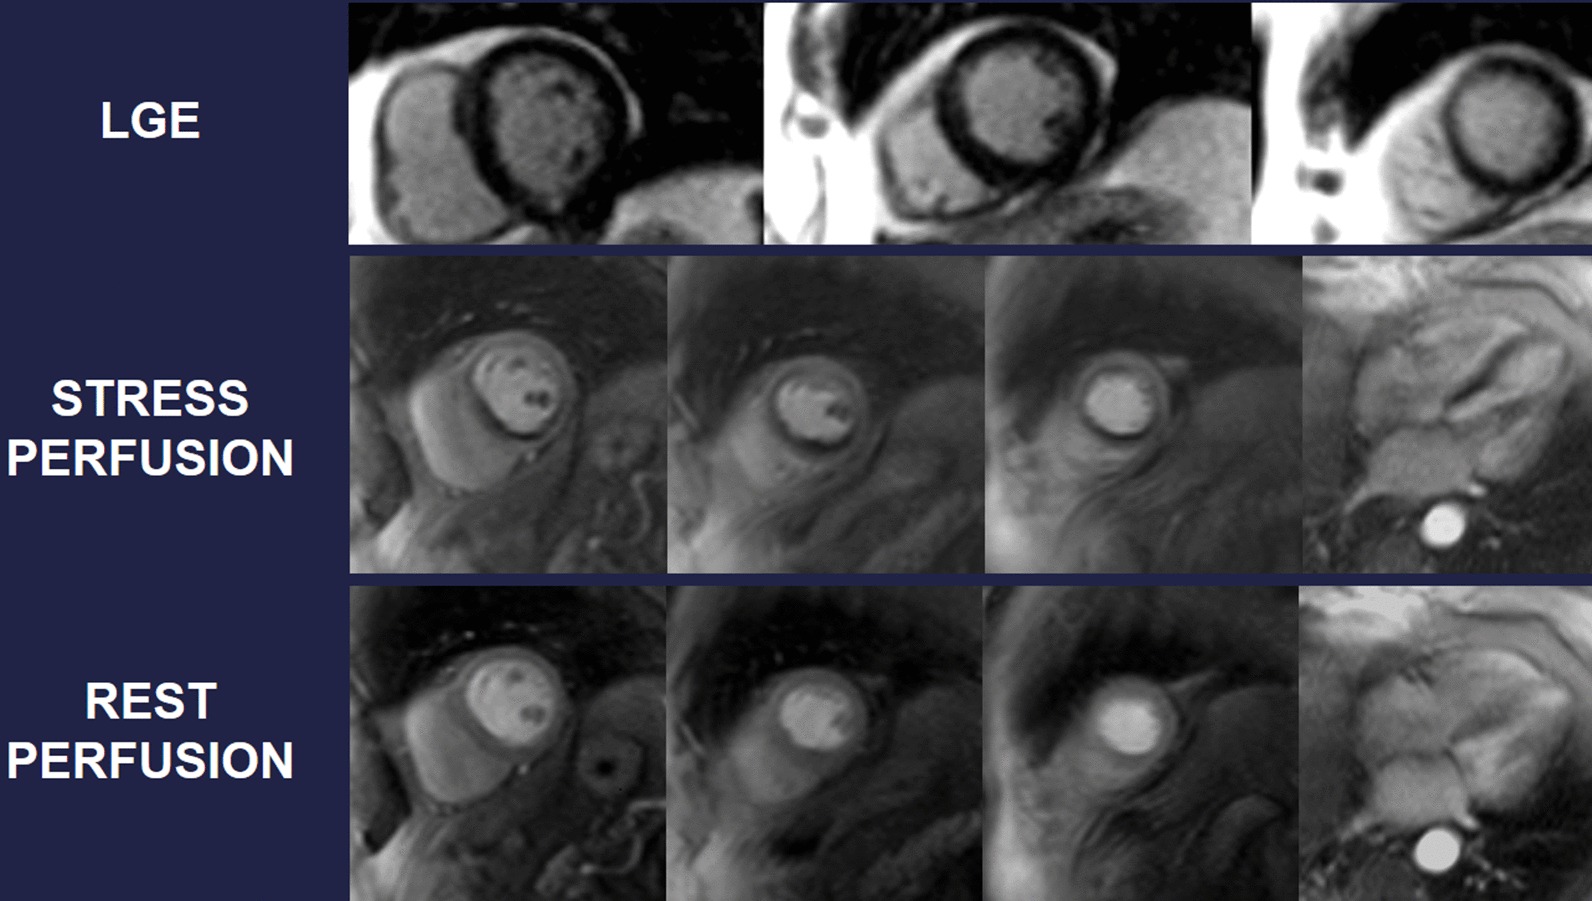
A 75 year-old male with hypertension and hyperlipidemia reported exertional dyspnea during rehabilitation post-stroke. Transthoracic echocardiography (TTE) showed mild left ventricular (LV) systolic dysfunction, and he had difficulty walking. Given concern for myocardial ischemia, he was referred for CMR with stress. Late gadolinium enhancement (LGE) demonstrates a small subendocardial infarct in the basal inferior wall, while myocardial perfusion acquired during adenosine infusion (stress) shows extensive perfusion abnormality that mostly resolves on resting perfusion imaging. Cine CMR demonstrates mild segmental LV dysfunction. These findings prompted invasive coronary angiography that showed high-grade multivessel coronary artery disease (CAD); post-revascularization, functional capacity improved
